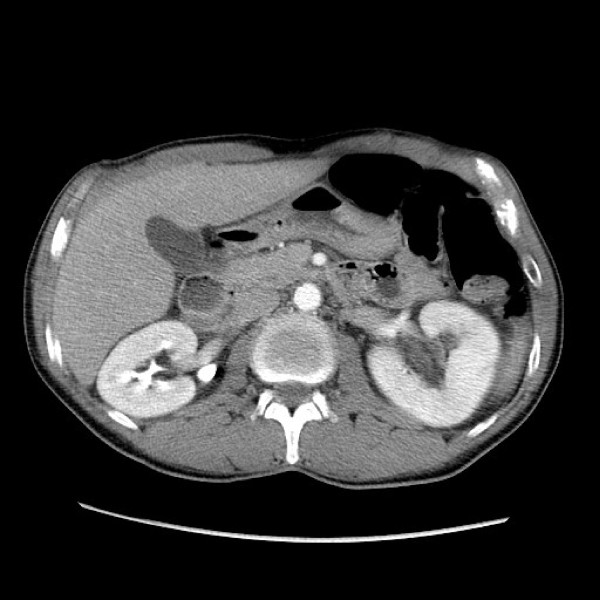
CT scan of Case 1: bladder cancer mass with associated left hydronephrosis and hydroureter.
